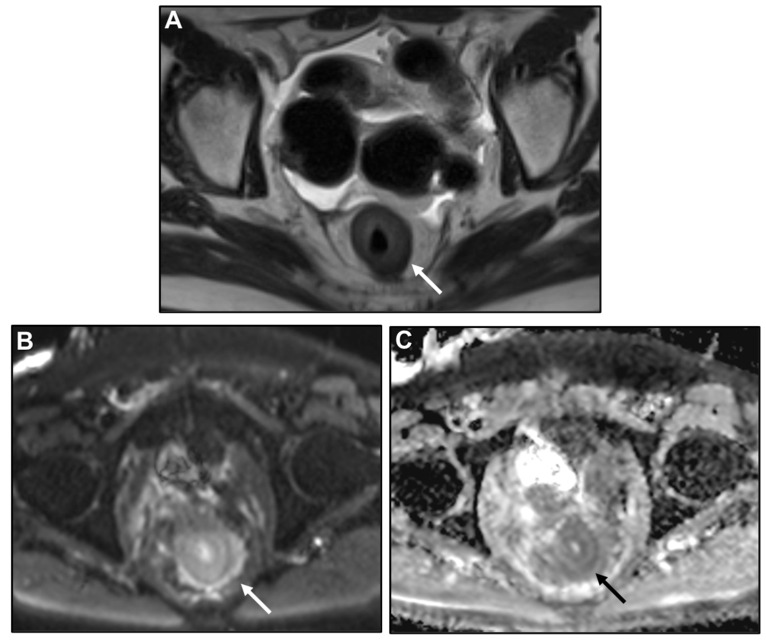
Concentric ring pattern on T2-weighted imaging (T2WI) and diffusion-weighted imaging (DWI). (A) MR image: Axial T2WI, (B) and (C) MR image: DWI and corresponding apparent diffusion coefficient (ADC). Shown is linitis plastica-like rectal metastasis from breast adenocarcinoma. Linitis plastica-like metastatic infiltration presents as mural thickening with preserved zonal anatomy. The stratified layers remain visible and their distinction appears accentuated. On both T2WI and DWI, the hallmark feature is a concentric ring pattern (arrow).
